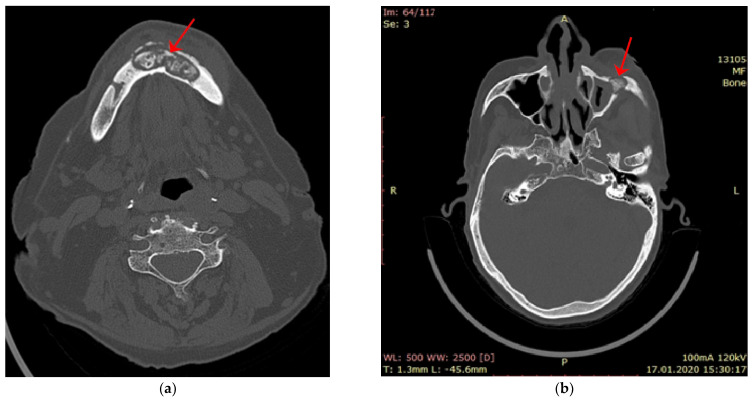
CT image: (a) mandibular axial section that shows a bone sequestrum in the mandibular arch region, radio-transparency (osteolysis), which surrounds a radio-opacity area; (b) maxillary axial section that shows a sequestrum in the left zygomatic bone, radio-transparency (osteolysis), which surrounds a radio-opacity area.
